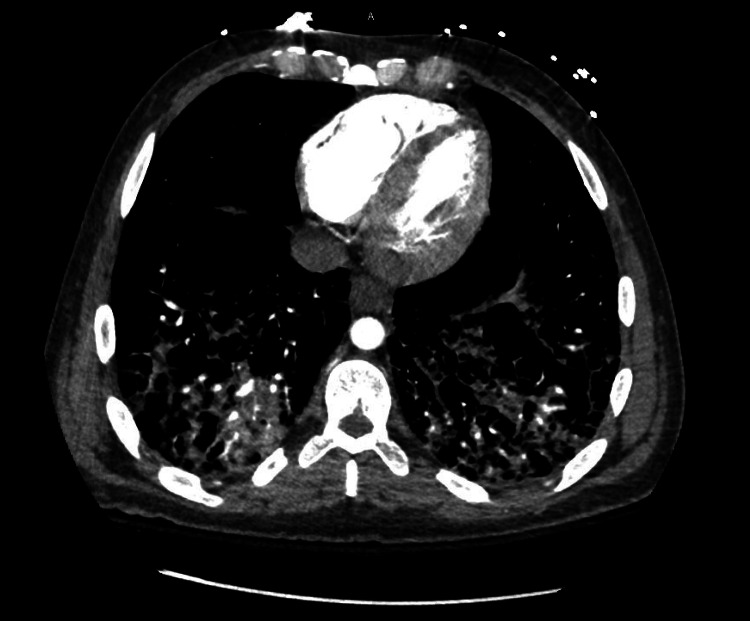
CT of the thorax after initial episode of desaturation, which was negative for any perfusion defects but did show ground-glass opacities.
